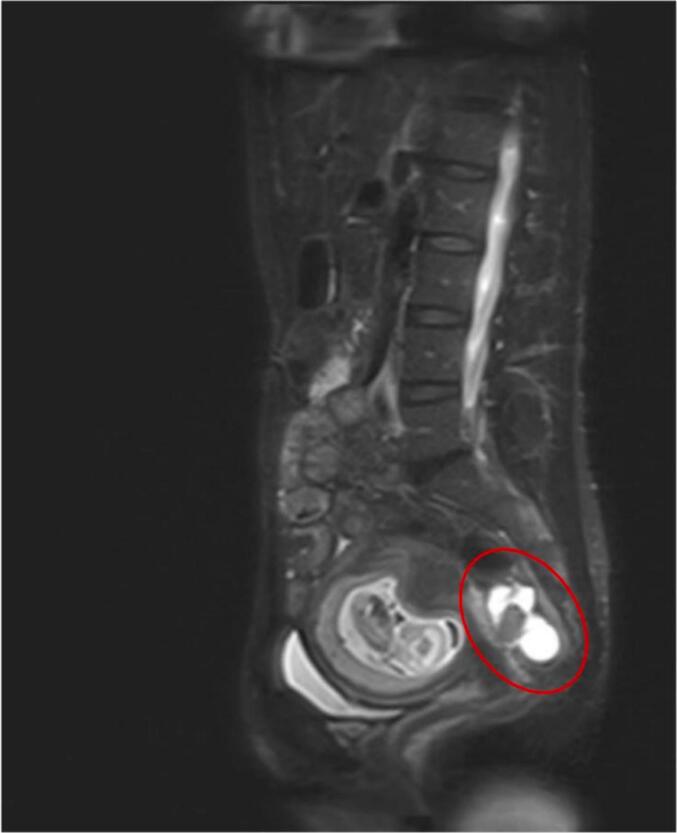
I) July 2018, T2-weighted magnetic resonance imaging of the pelvis demonstrating a pararectal lesion (red circle) with close contact to the rectum, consistent with suspected recurrent adult granulosa cell tumor.
